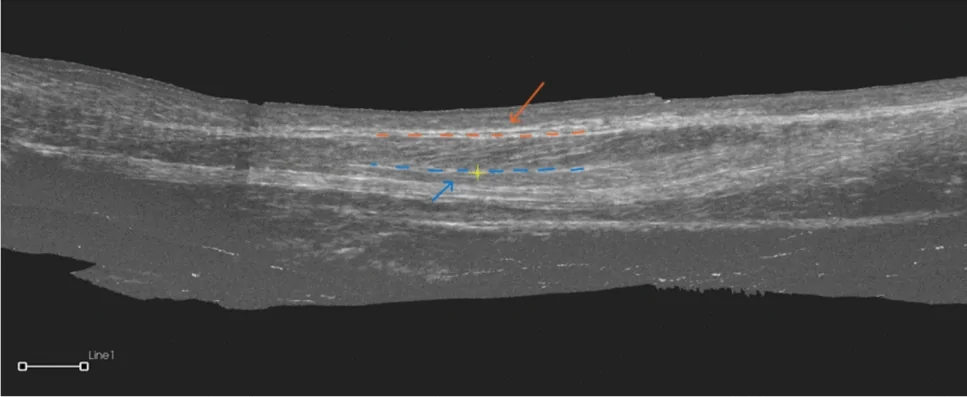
Longitudinal ultrasound image of the biceps femoris long head (BFlh) showing the mid-belly region (50% of muscle-belly length) analyzed with Method 1, in which the imaging plane was oriented perpendicular to proximal aponeurosis. The distal aponeurosis (orange dashed line) and proximal aponeurosis (blue dashed line) are indicated, and the yellow marker identifies the fascicle attachment point at the midpoint of the proximal aponeurosis. Proximal is to the left and distal to the right. Scale bar = 2 cm (20 mm)
